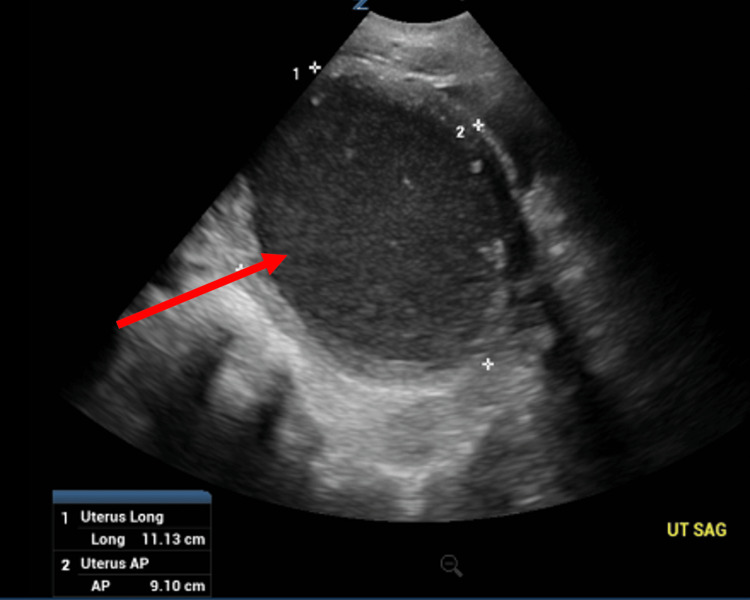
Complete pelvic ultrasound; view of the uterus.
